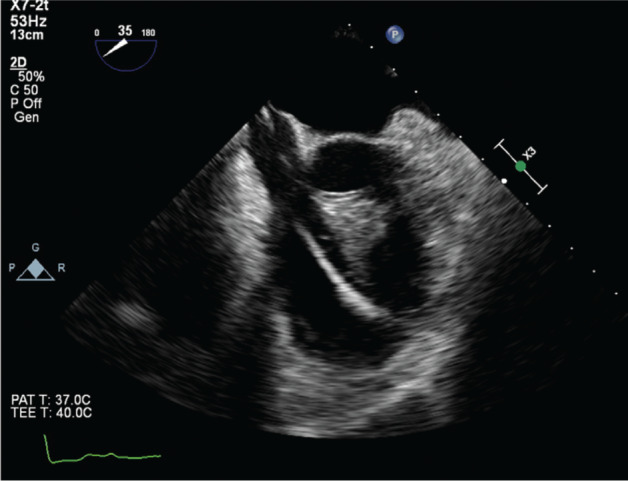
Transesophageal echocardiogram revealing a 3.2-cm × 2.4-cm mass attached to the RV lead, extending to the RV free wall.
